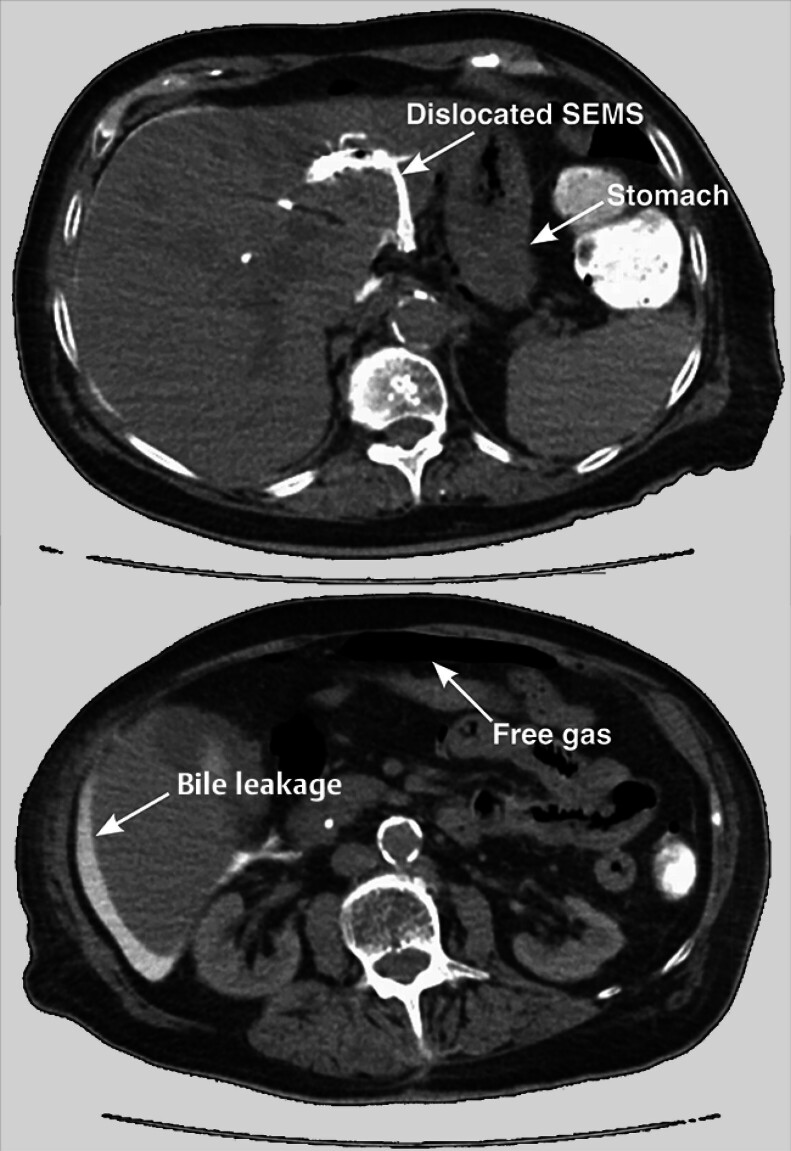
Computed tomography scan showed a dislocated self-expanding metal stent from the endoscopic ultrasound-guided hepaticogastrostomy (EUS-HGS), bile leakage, and pneumoperitoneum.
